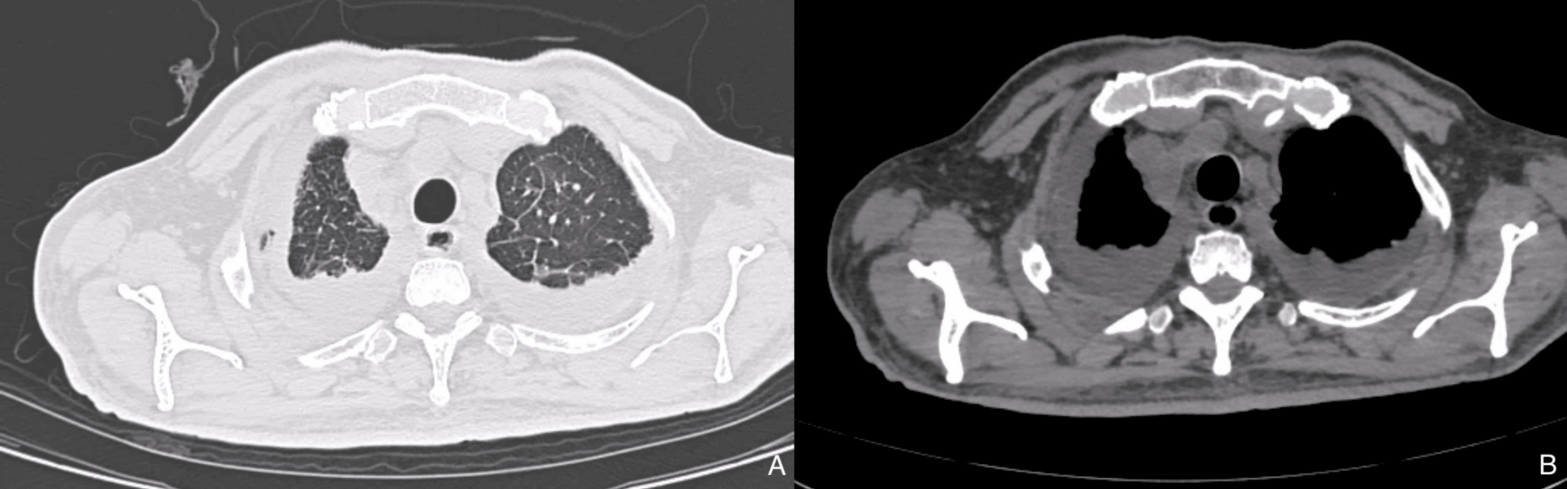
HRCT chest shows moderate bilateral pleural effusion and areas of interlobular septal thickening HRCT: High-resolution computed tomography, A: Lung window, B: Mediastinal window
